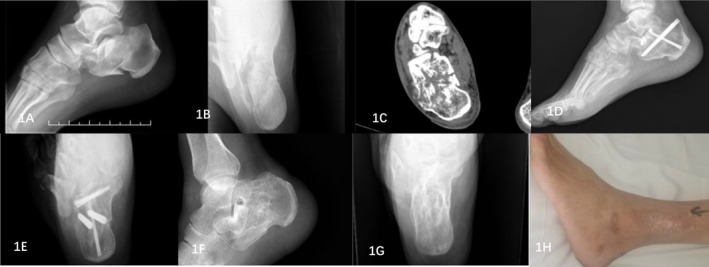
Imaging data of a typical PMISIF patient. (A) Preoperative calcaneal lateral x‐ray image showing that the articular surface of the calcaneus collapsed. (B) Preoperative axial x‐ray of the calcaneus revealed that the width of the calcaneus increased and that the length of the calcaneus shortened. (C) Preoperative MR image of the calcaneal fracture suggested a Sander type III fracture. (D) Postoperative calcaneal lateral x‐ray image showing calcaneal height recovery. (E) Postoperative calcaneal axial x‐ray image showing that the width and length of the calcaneus had recovered. (F) Lateral x‐ray films of calcaneus after removal of internal fixator showed calcaneus recovery. (G) Axial x‐ray films of calcaneus after removal of internal fixator showed calcaneus recovery. (H) The wound healed well after the operation.
